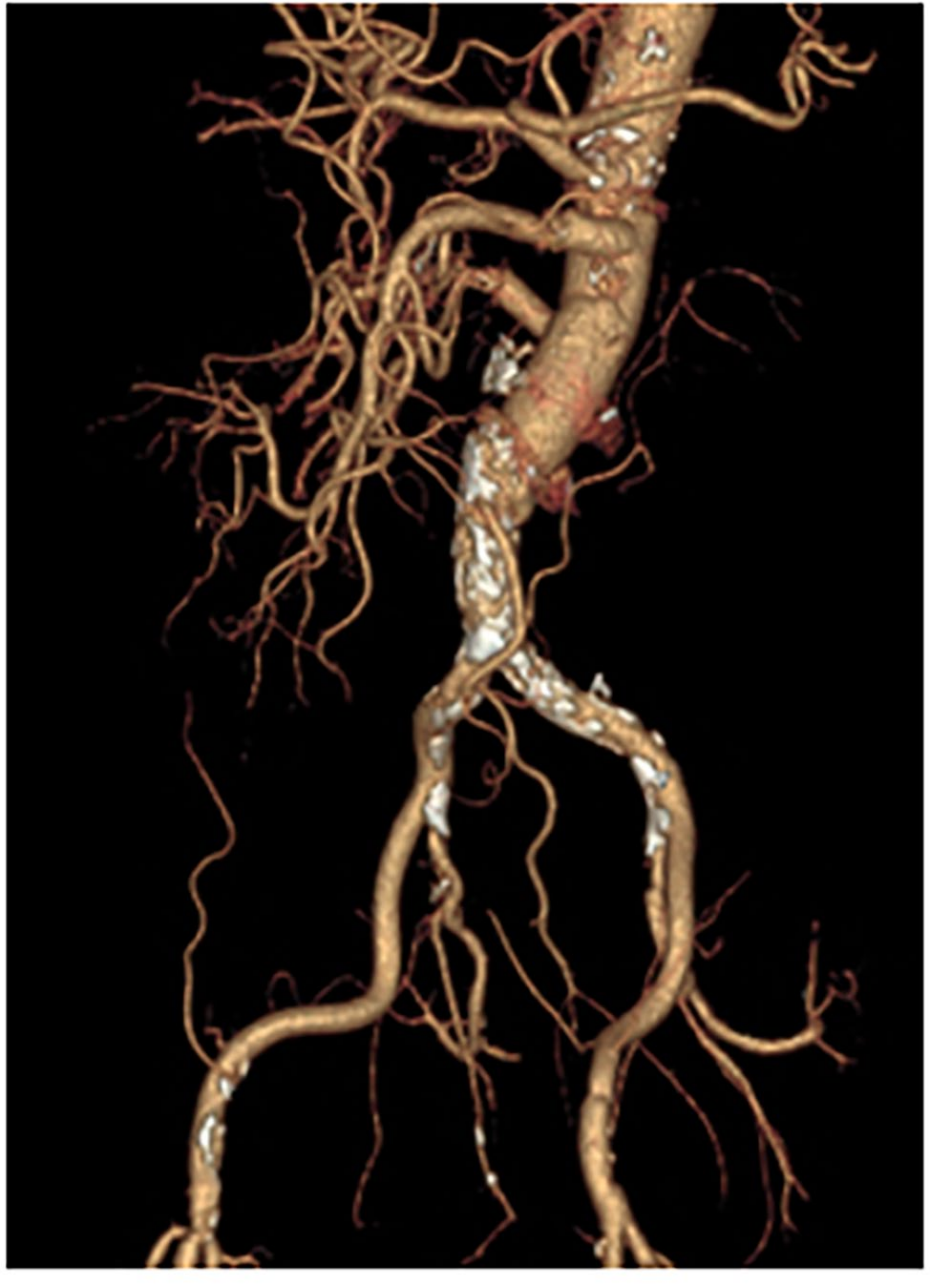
Computed tomography (CT) (5 years) after the second operation shows no pseudoaneurysm of the anastomosis. However, the graft to the left renal artery was occluded
